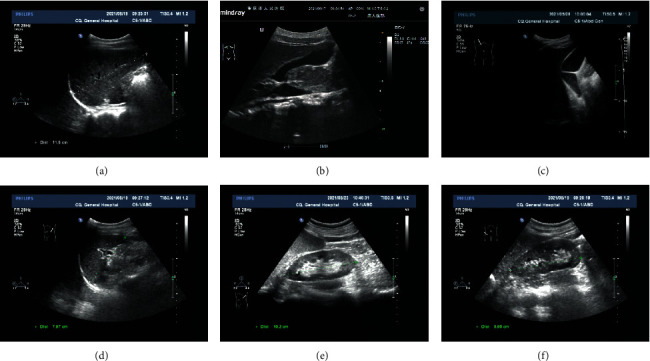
Ultrasound findings of the liver, gallbladder, spleen, and kidney of one subject. (a–f) Ultrasound examination for liver span (a), clear echoes (b), and cholestasis (c) in the gallbladder, spleen length (d), left kidney length (e), and right kidney length (f).
